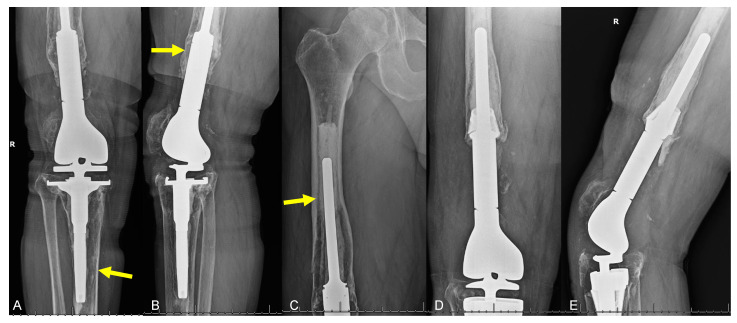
Imaging of 64-year-old male diagnosed with chondrosarcoma of the distal femur treated with resection and endoprosthetic reconstruction, presenting 13 years after reconstruction with knee pain. (A) AP and (B) lateral radiographs show aseptic loosening (type 2 failure; yellow arrows), with (C) extension to the femoral stem. (D) AP and (E) lateral radiographs taken post-revision with metaphyseal cones and impaction bone grafting reveal well-seated endoprosthesis without significant peri-prosthetic lucencies.
